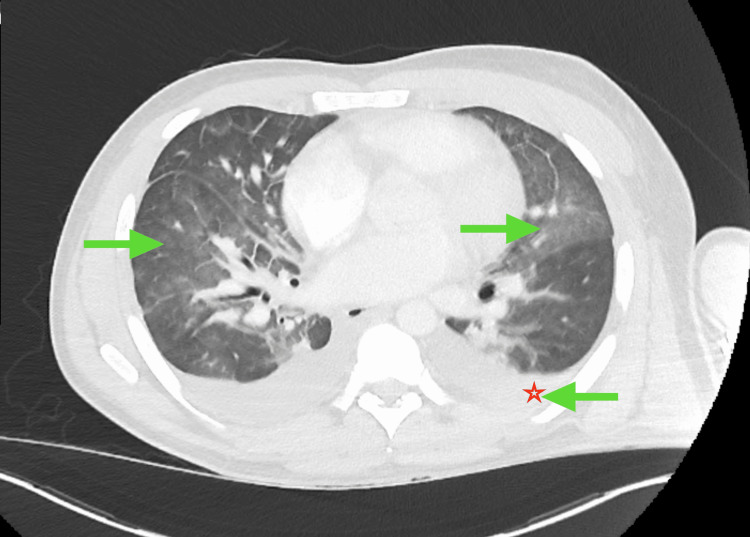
Chest CT scan with and without contrast, axial view, showing bilateral pulmonary infiltration (green arrows) and pleural effusion (green arrow and star)
